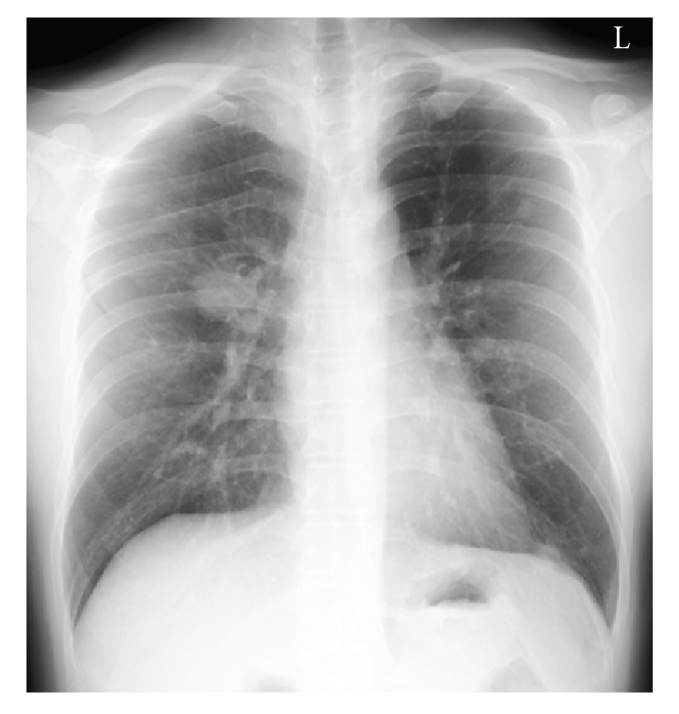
Plain radiography of lung. Multiple metastatic lesions in both lungs were found at first visit.
